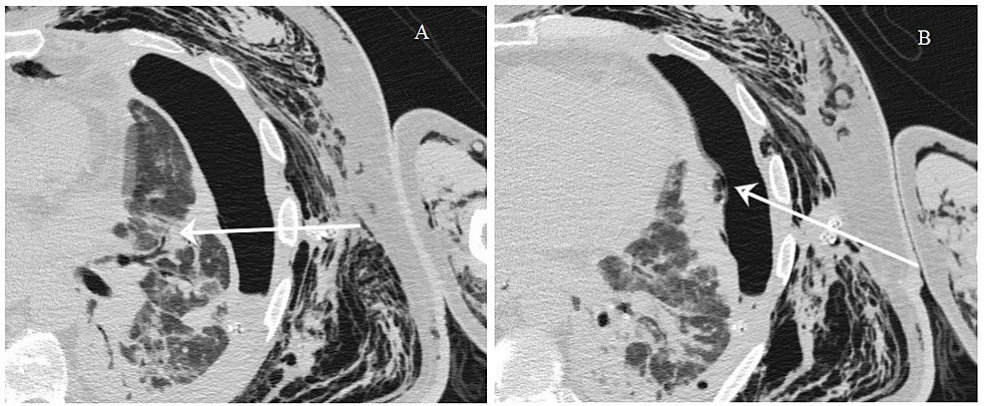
CT of the chest showing the bronchopleural fistulous tract. (A) Arrow indicating the fistula originating from a small bronchus. (B) Arrow indicating the fistula terminating in the pleura, causing the pneumothorax.
